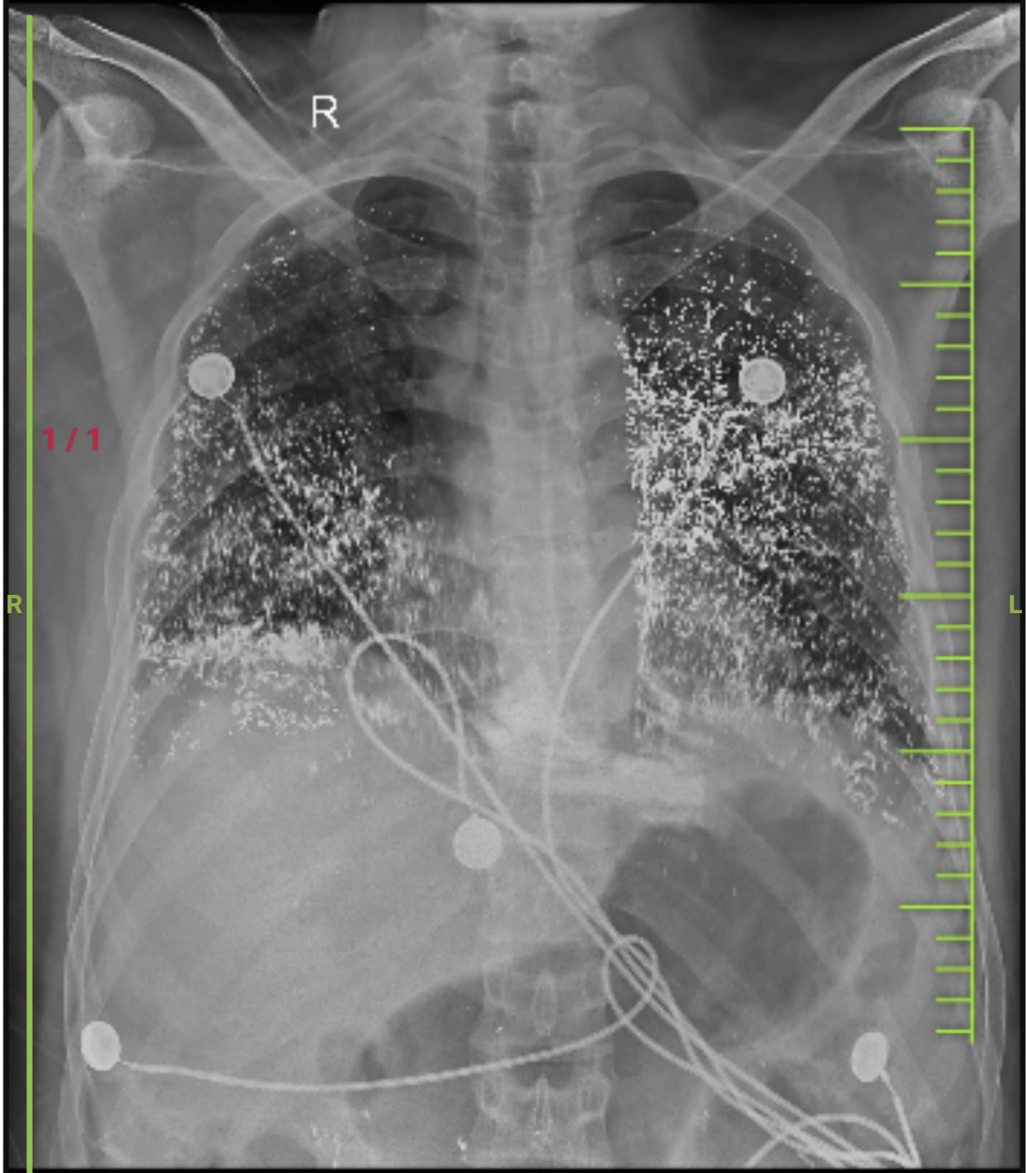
X-ray of patient showing linear radio-opacities in bilateral lung fields
